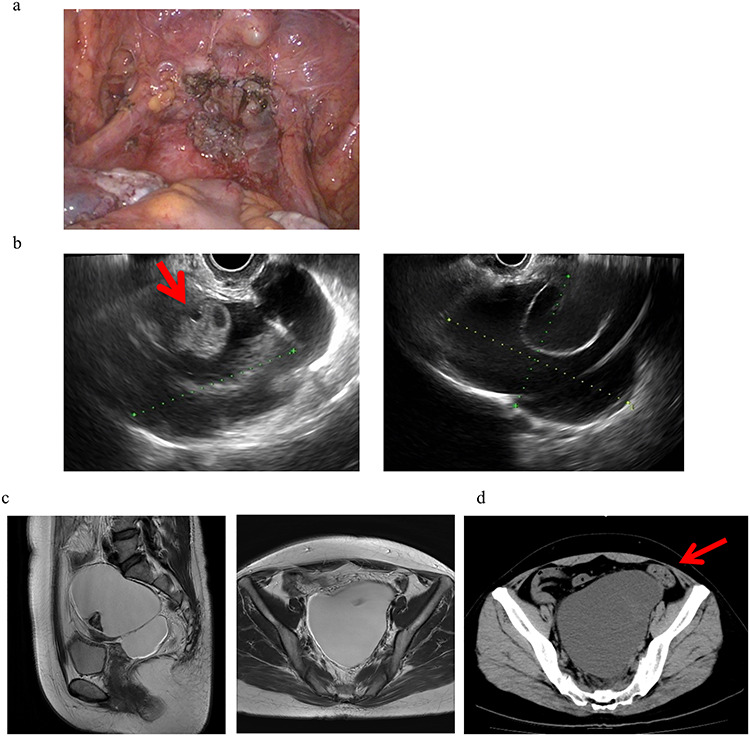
Clinical findings of case 1. (a) Findings at the end of TLH. No abnormal findings were detected. (b) An US image of PIC in case 1. An ovary with a normal appearance was observed within the multilocular cyst. (c) T2-weighted MRI image. (d) CT findings with abdominal pain. A giant cyst appears to compress the sigmoid colon, resulting in abdominal pain and the impaired passage of the stool mass.
